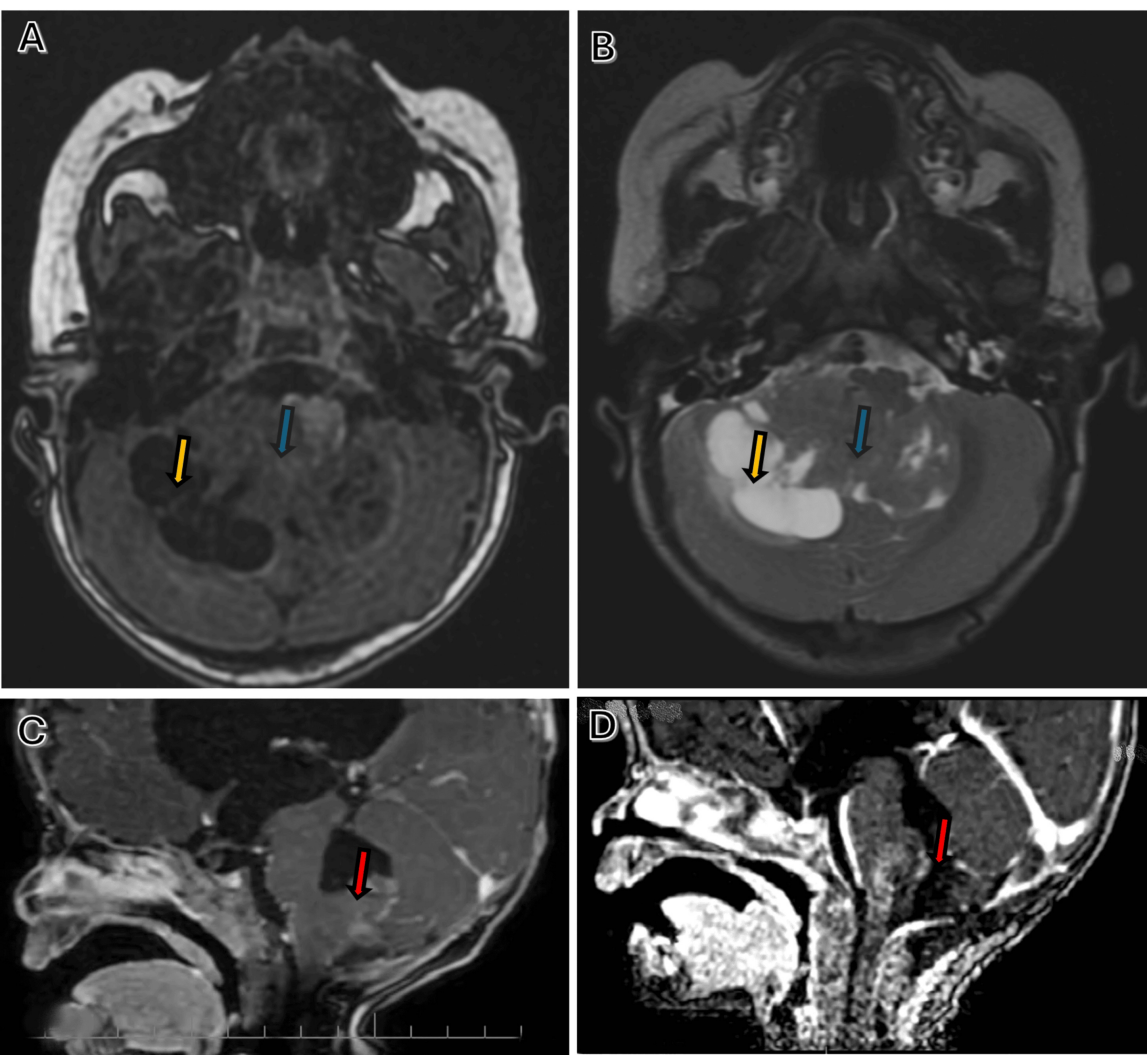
MRI of the posterior fossa tumor Axial T1-weighted (A) and T2-weighted (B) MRI sequences demonstrate a heterogeneous posterior fossa mass with mixed solid (blue arrow) and cystic (yellow arrow) components, arising from the cerebellum and extending into the fourth ventricle with extension toward the CPA. Sagittal contrast-enhanced T1-weighted images show the preoperative appearance of the tumor (red arrow) (C) and the immediate postoperative status on postoperative day 1 (red arrow) (D), confirming partial tumor resection. MRI: magnetic resonance imaging, CPA: cerebellopontine angle
